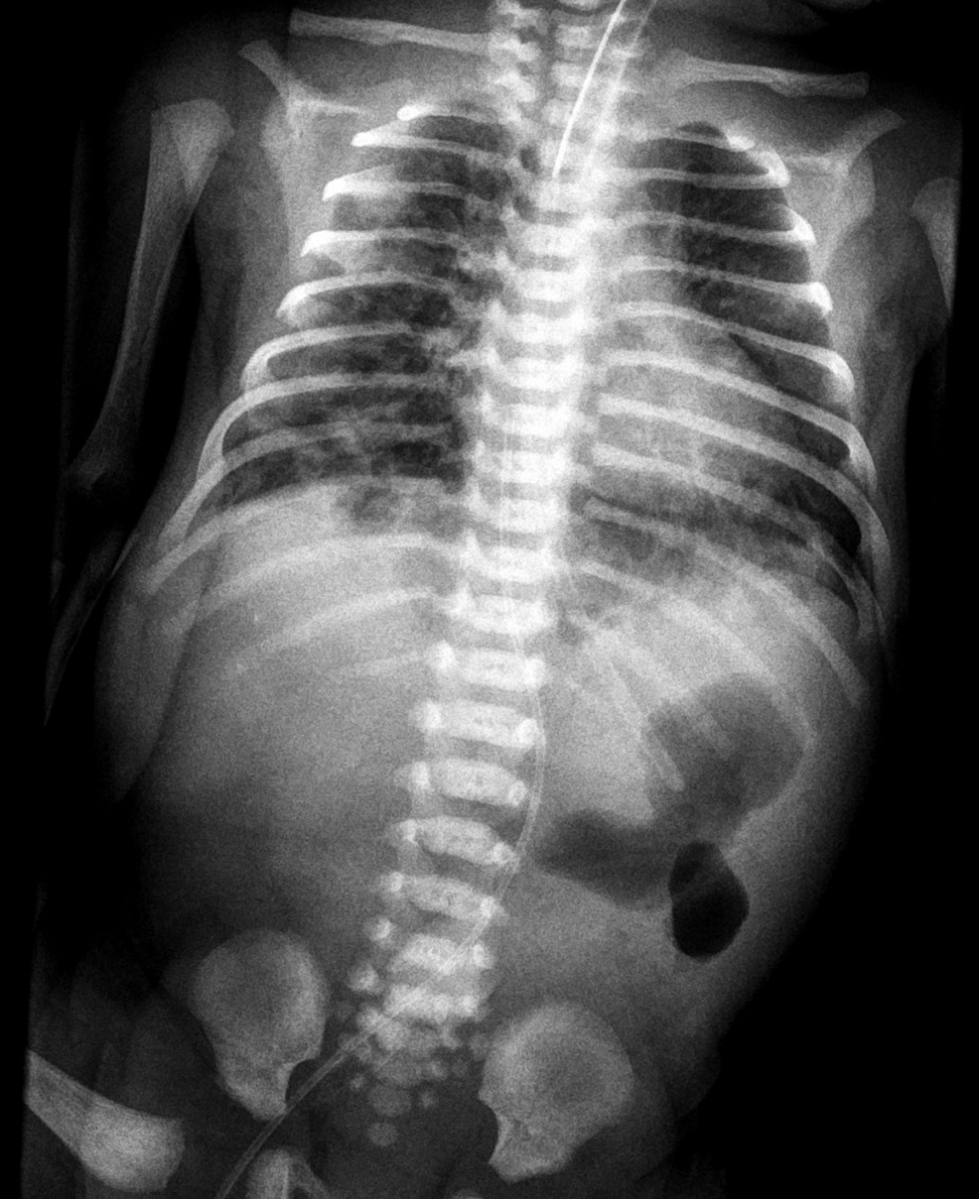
X-ray image post-delivery confirming the dilated loops.
